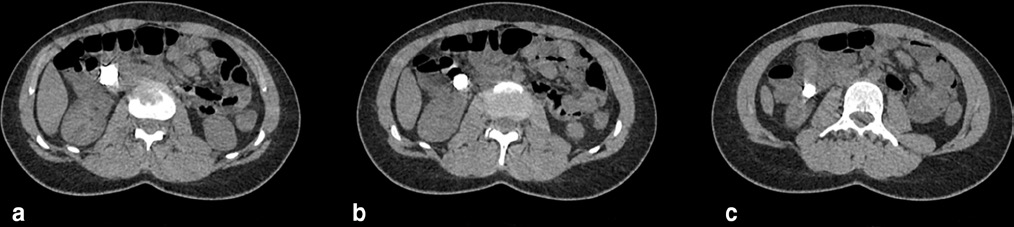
a,b,c: Sequential non-contrast axial CT images, demonstrating the aggregation of button magnets. Metal reduction artefact applied.
